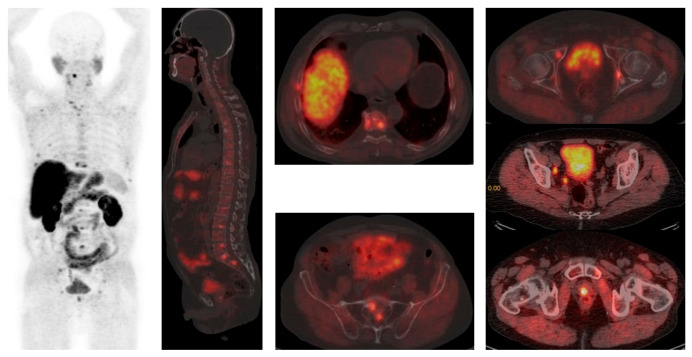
[^18^F]F-Choline PET/CT reveals widespread disease in bones (vertebrae, ribs, sacral bone) and parailiac lymph nodes. Courtesy of Ass Prof Sinisa Stojkovic, Clinical Institute of Nuclear Medicine and Thyroid Diseases, University Clinical Centre of the Republic of Srpska.
